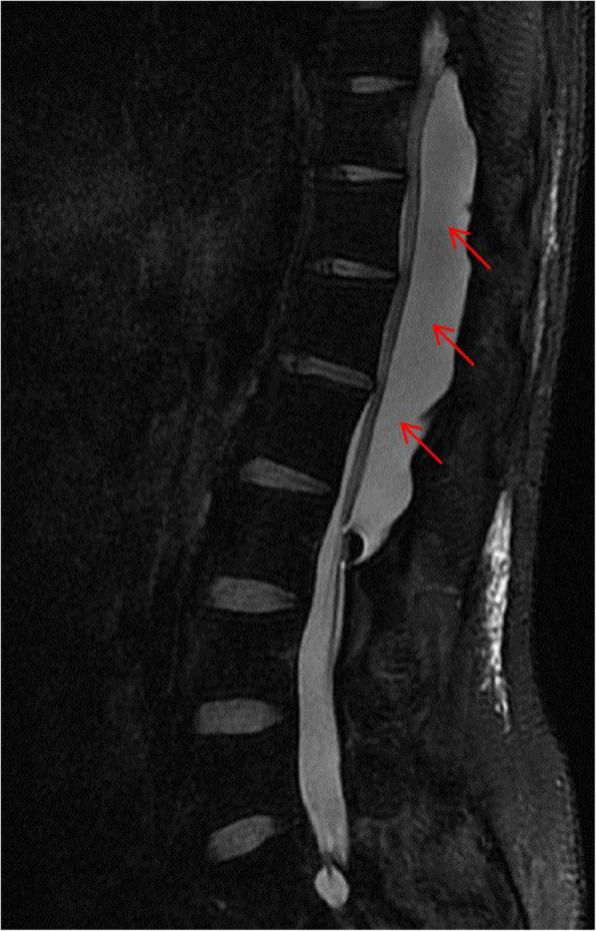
The magnetic resonance imaging (Sagittal T2-weighted) after 20 mL of fluid was aspirated from the cyst (red arrows)
